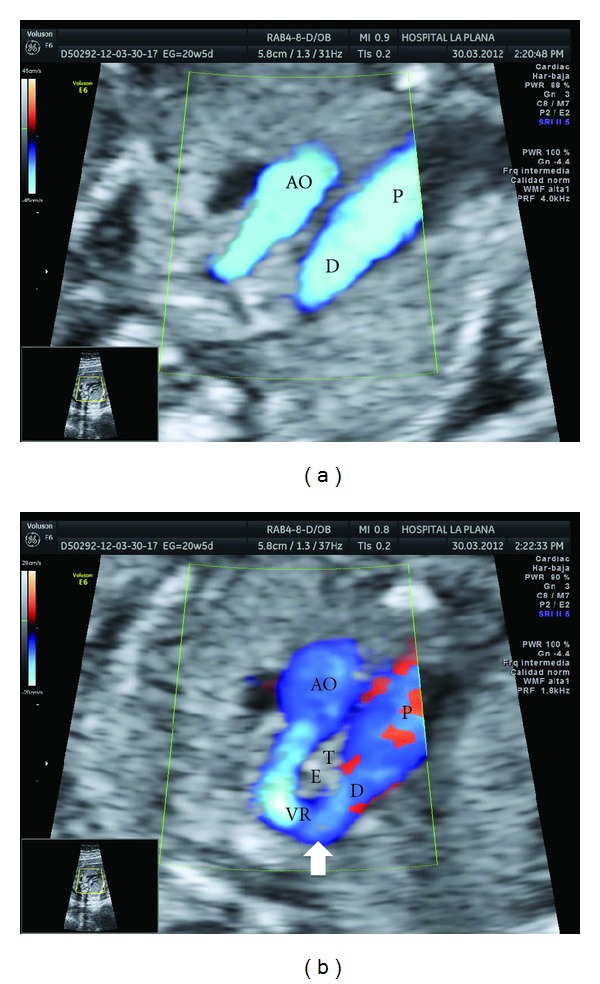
(a) Color Doppler ultrasound (three vessels and trachea view) showing two parallel vessels. This was the first clue to the diagnosis of cardiac malformation and subsequent fetal aneuploidy. AO: aortic arch, P: pulmonary vein, D: ductus arteriosus. (b) Color Doppler ultrasound showing a vascular retroesophageal ring formed with the union of the right aortic arch and the prolongation of the ductus arteriosus. As the aortic arch passes over the right bronchus, the encounter between both circulations occurs behind the esophagus. AO: aortic arch, P: pulmonary vein, D: ductus arteriosus, VR: vascular ring, T: trachea, E: esophagus. In the union of the ductus arteriosus and the aortic arch a small outpouching is seen forming a small diverticulum of Kommerell (arrow), where the aberrant left subclavian artery (not seen in this picture) joined.
